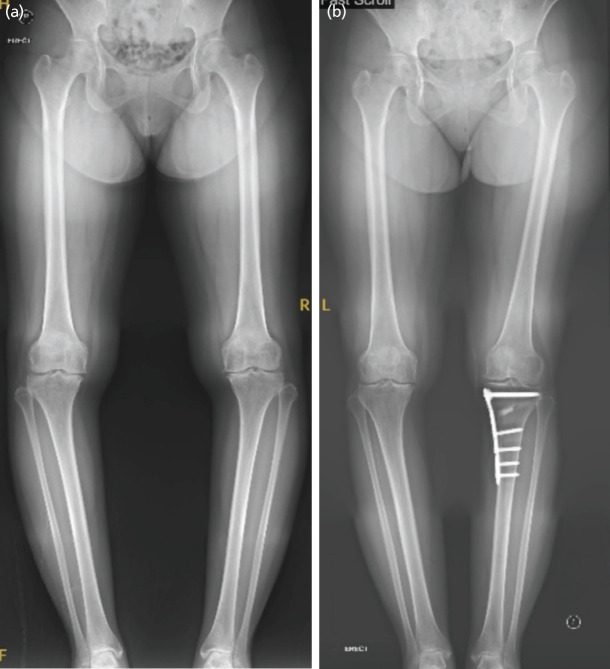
(a) Pre-operative and (b) one-year post-operative left MOWHTO long leg alignment radiographs with a Tomofix plate and Chronos.
